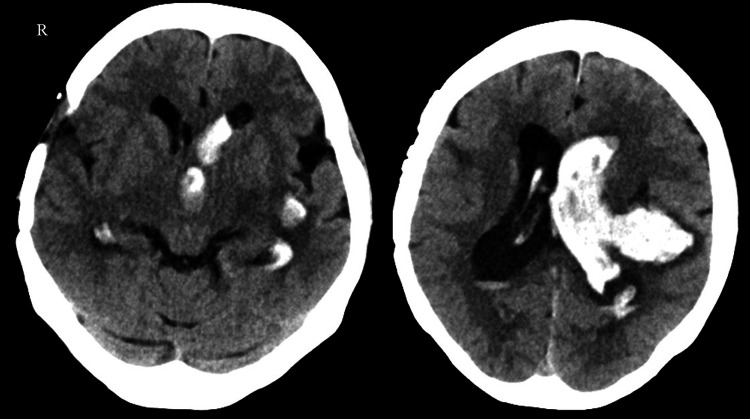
The patient's head CT on admission revealed a hemorrhage in the left lateral ventricle, leading to the diagnosis of cerebral hemorrhage associated with moyamoya disease. R indicates the right side.
